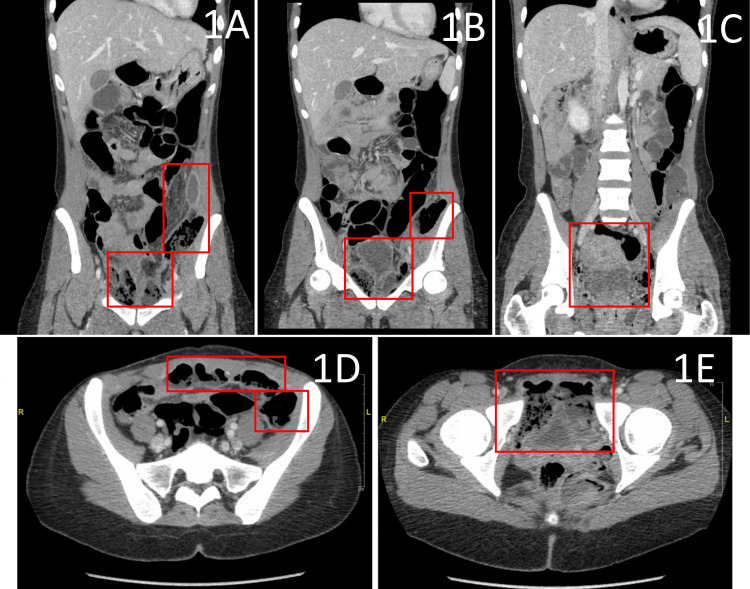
CT imaging of the abdomen and pelvis with IV contrast. Coronal images demonstrate extraluminal air and abscess pockets in the left-sided perineum (A-C), with extension along the left lateral pelvic reflection (B), indicated by the red boxes. Axial CT imaging evidences abscess extension into the anterior intra-abdominal wall (D) extending from the underlying perineal abscess cavity (E), also indicated in red. Extraluminal air pockets and possible stool were initially interpreted as concerns of bowel perforation, providing an indication for emergent surgical intervention.
